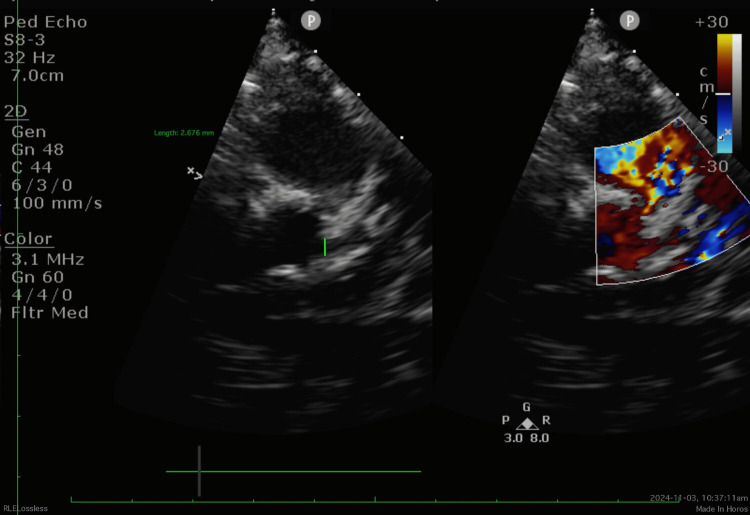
Echocardiography showed diffuse left main coronary artery (LMCA) dilatation measuring 2.7 mm (Z score +3.65)
